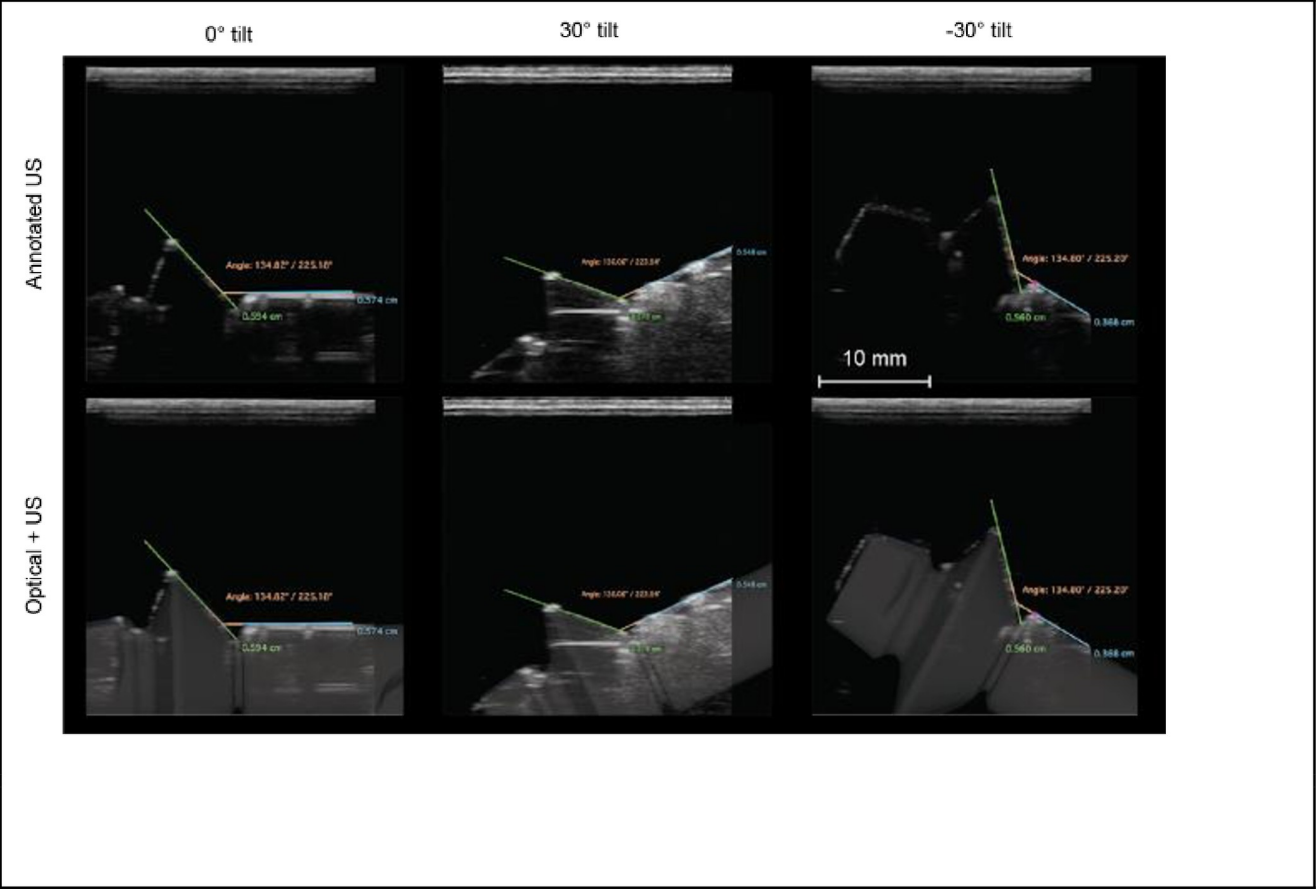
Top row: Measurement of the abutment angle on abutment 9–3. Columns left, middle and right, are labeled with the degree angle between the abutment and the ultrasound transducer, i.e., 0°, +30°, and -30° with respective abutment-holder angle measurements of 134.8°, 136.1°, and 134.8°. Bottom row: Overlay images for the respective optical (reference) and ultrasonic scans. (Scale bar for all images).
